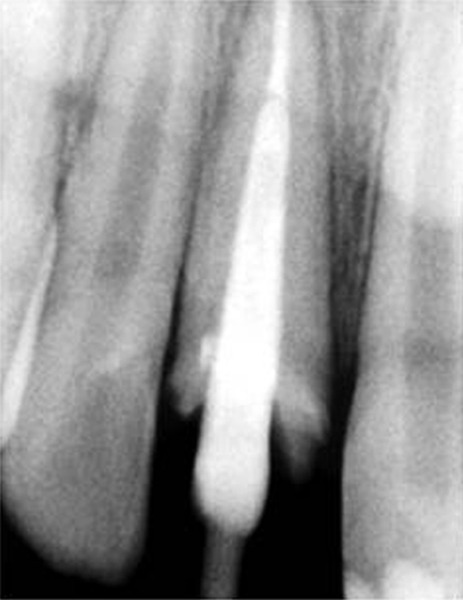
Radiographic confirmation of the custom post
